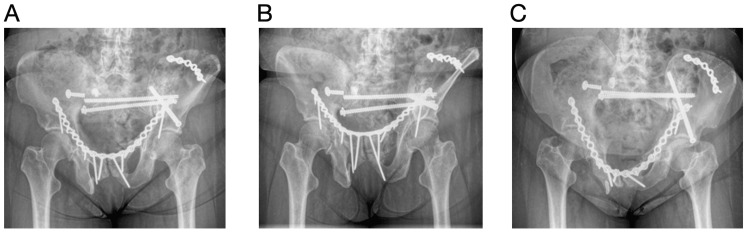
Two month post op radiographs Images A (AP pelvis) , B (pelvic outlet), and C (pelvic inlet) demonstrate the broken S1 transsacral screw and broken plate anteriorly.
